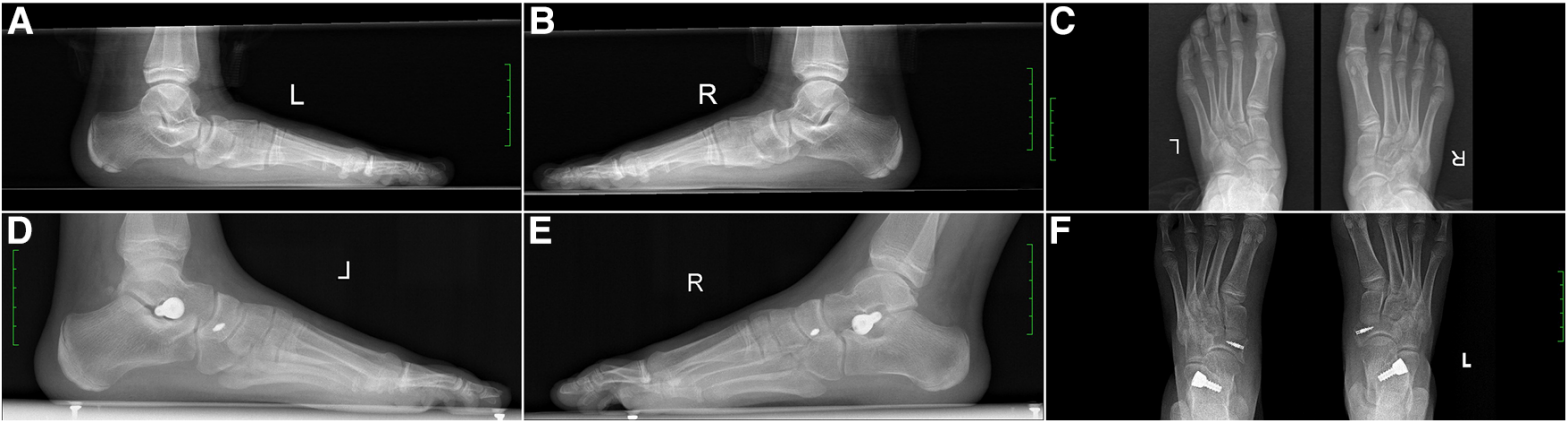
An 10-year-old female patient with bilateral type II painful accessory navicular (AN) combined with flexible flatfoot underwent a modified Kidner surgery combined with subtalar arthroereisis tarsal screw implantation. (A) Lateral x-ray images of left feet before surgery: Meary's angle:30, APTMT: 16, Talar-second metatarsal angle: 23, Pitch angle: 16, TCA: 56, Calcaneal angle: 37, Talar tilt angle: 40, LTCA: 20. (B) Lateral x-ray images of right feet before surgery: Meary's angle: 20, APTMT: 13, Talar-second metatarsal angle: 20, Pitch angle: 18, TCA: 55, Calcaneal angle: 30, Talar tilt angle: 36, LTCA: 13. (C) AP x-ray images of both feet before surgery. (D) Lateral x-ray images of left feet 1-year postoperative follow-up: Meary's angle: 5, APTMT: 13, Talar-second metatarsal angle: 10, Pitch angle: 20, TCA: 43, Calcaneal angle: 35, Talar tilt angle: 23, LTCA: 11. Parameters shows good positioning of the anchor screw and tarsal screw and good alignment of foot arch. (E) Lateral x-ray images of right feet 1-year postoperative follow-up: Meary's angle: 2, APTMT: 1, Talar-second metatarsal angle: 3, Pitch angle: 14, TCA: 33, Calcaneal angle: 36, Talar tilt angle: 20, LTCA: 5. Parameters shows good positioning of the anchor screw and tarsal screw and good alignment of foot arch. (F) AP x-ray images of both feet 1-year postoperative follow-up, showing correction of AN deformity in both feet.
